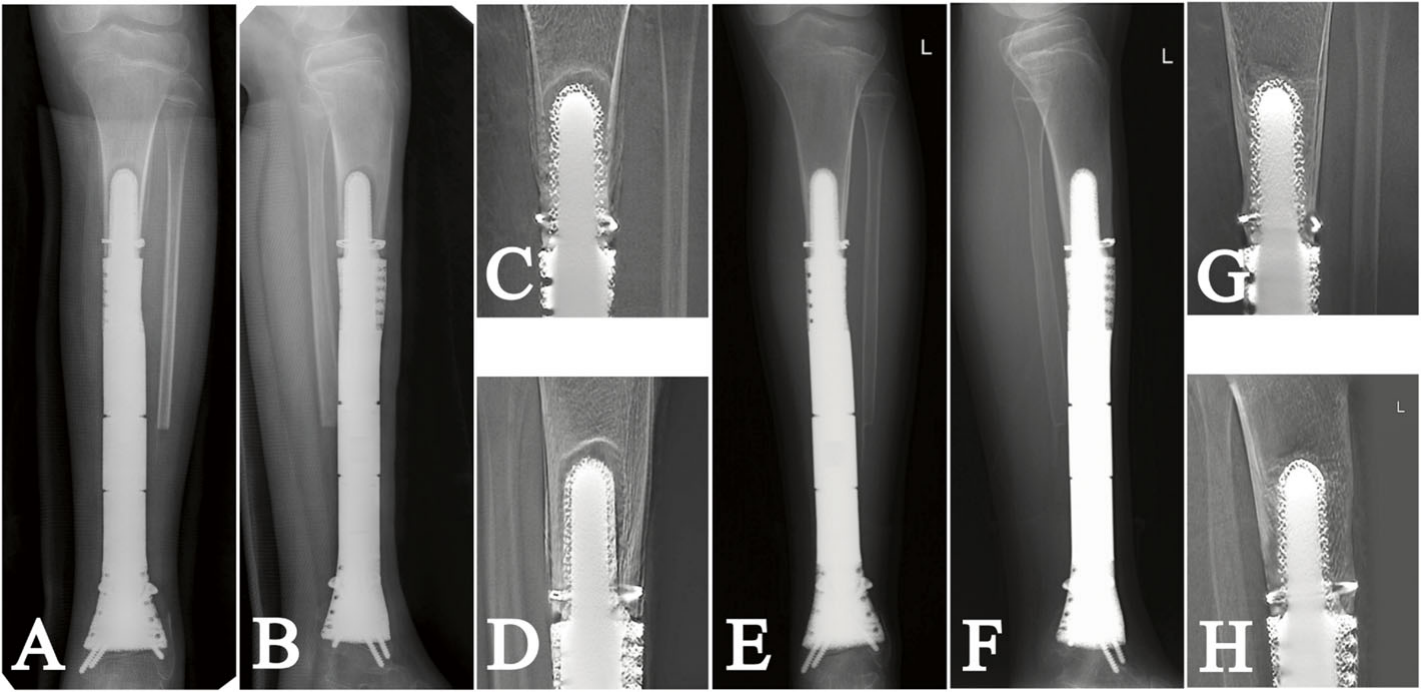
The A AP and B lateral plain radiographs show early aseptic loosening in patient 9 3 months postoperatively; The C AP and D lateral digital tomosynthesis graphs show radiolucency and hardened area around the stem. The E AP and F lateral plain radiographs and G AP and H lateral digital tomosynthesis images show well osseointegration at latest follow-up
